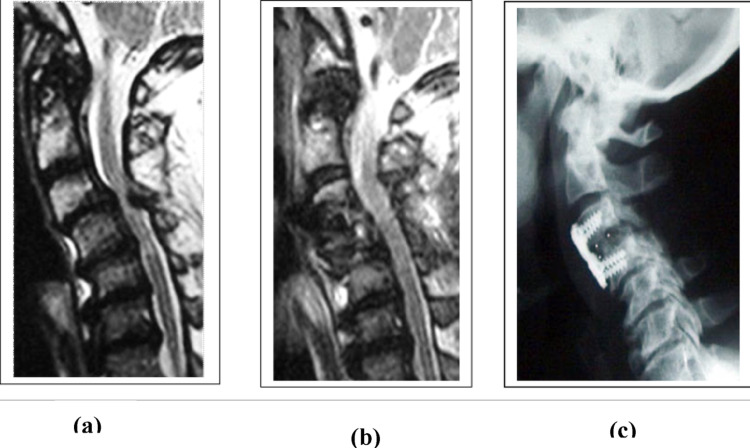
Representative radiological images of cervical spondylotic myelopathy with severe spinal cord compression. Preoperative sagittal T2-weighted MRI demonstrating marked anterior and posterior spinal cord compression at the C3–C4 level with associated intramedullary signal changes (a). Postoperative sagittal T2-weighted MRI following anterior cervical discectomy and fusion + posterior decompressive laminectomy, showing adequate spinal cord decompression (b). Postoperative lateral cervical spine X-ray demonstrating anterior fusion and alignment following instrumented fixation (c)
